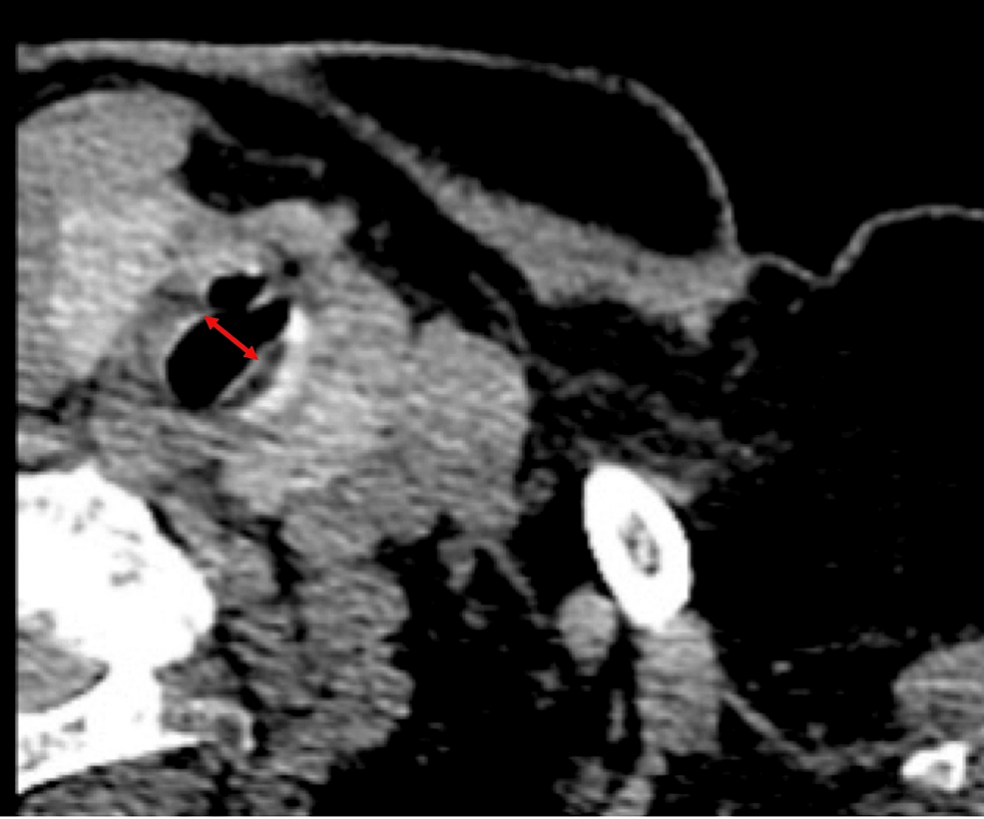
SGS on the preoperative CT image. The red arrow in the CT image shows the site of the SGS. The diameter of the SGS was approximately 7 mm. SGS: subglottic stenosis, CT: computed tomography
